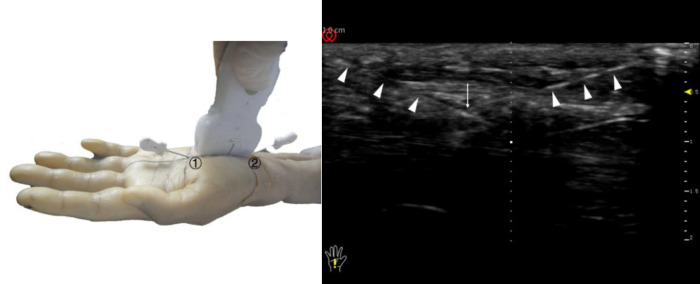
Ultrasound guided needle knife release technique completed (left) and ultrasound long axis display (right). The triangle refers to the needle knife position. The long arrow points to the intersection of the needle points of the needle knife. ①, needle entry point 1; ②, needle entry point 2.
